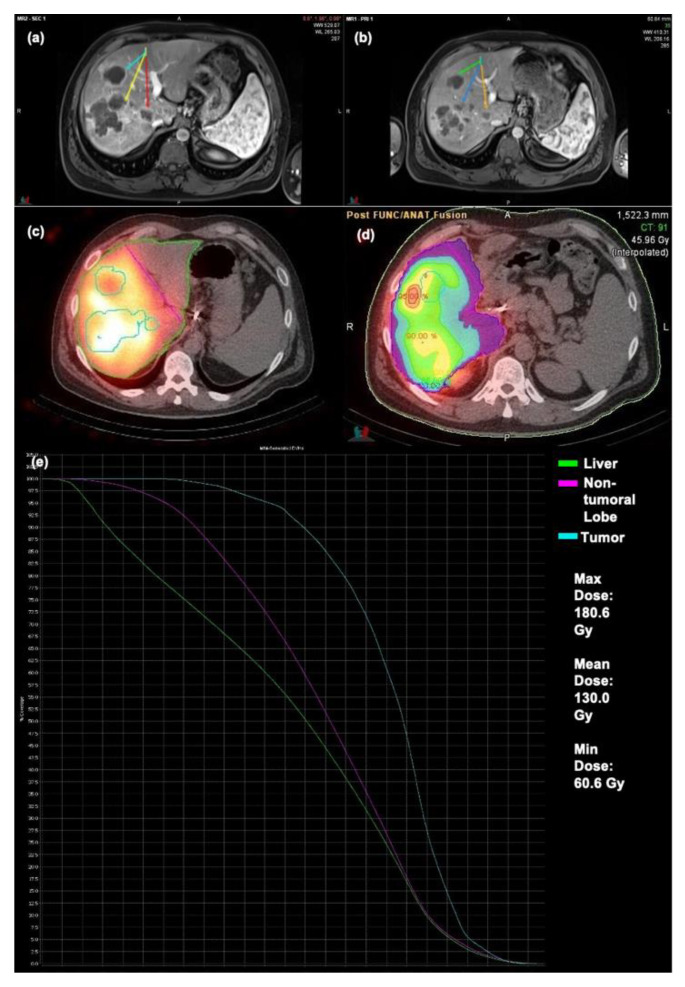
Patient exhibiting OR to Y90 therapy (a) Pre-op MRI (b) Post-op MRI (c) Bremsstrahlung SPECT/CT (d) Dosimetry map (e) DVH Curve.
